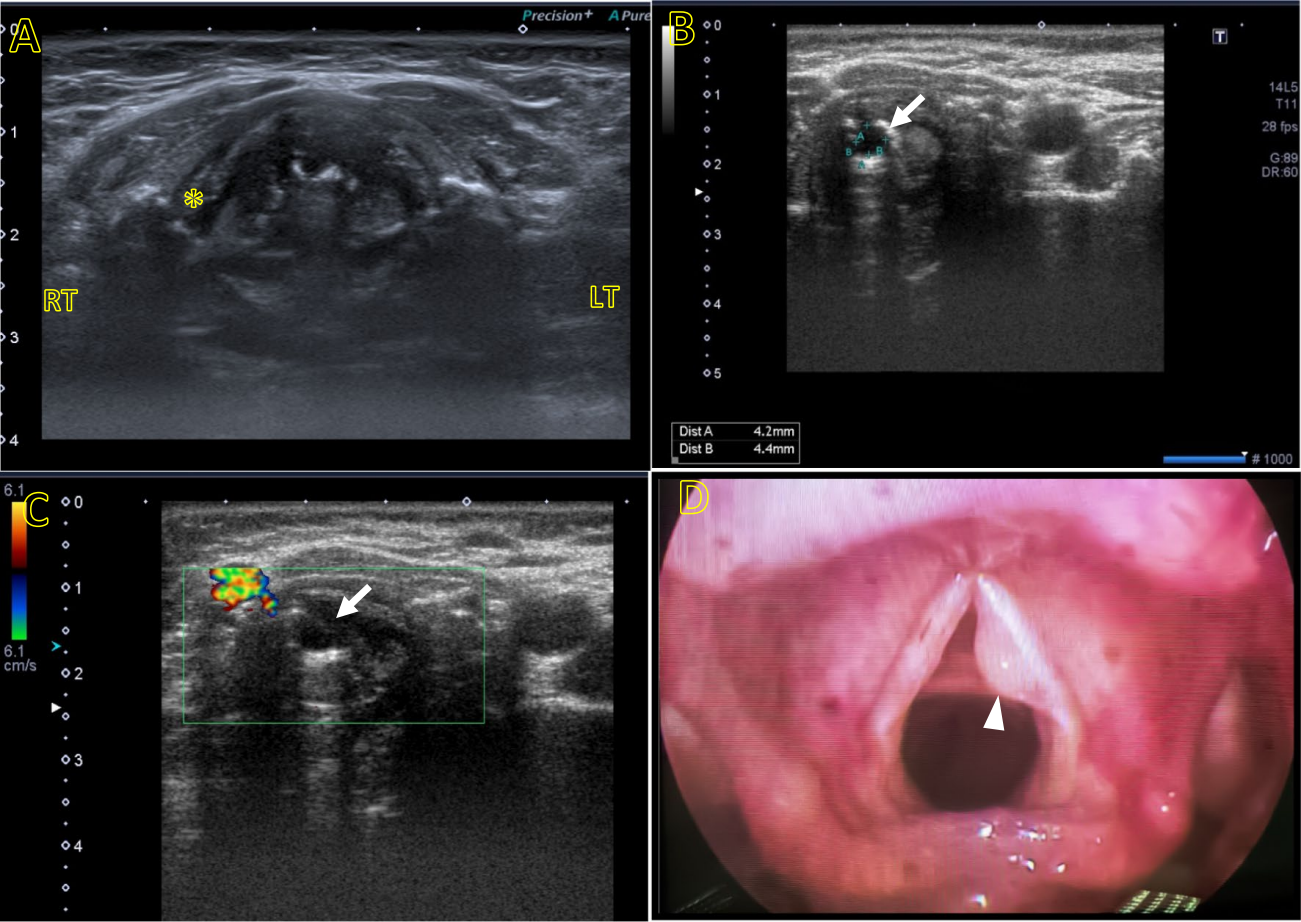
A 55-year-old female patient presented with hoarseness of voice and GERD. A Laryngeal ultrasound anterior axial view showing the anatomical landmarks along the level of the true vocal cord with thyroid cartilage seen (asterisk) and right/left landmarks on the image. B Left lateral axial view shows left VC anechoic lesion measures about 4 mm (arrow) with no color Doppler signal uptake could be traced C denoting vocal cord cyst. D Flexible laryngoscopy of the same patient shows a left VC cyst (arrow head), matching the sonographic diagnosis
